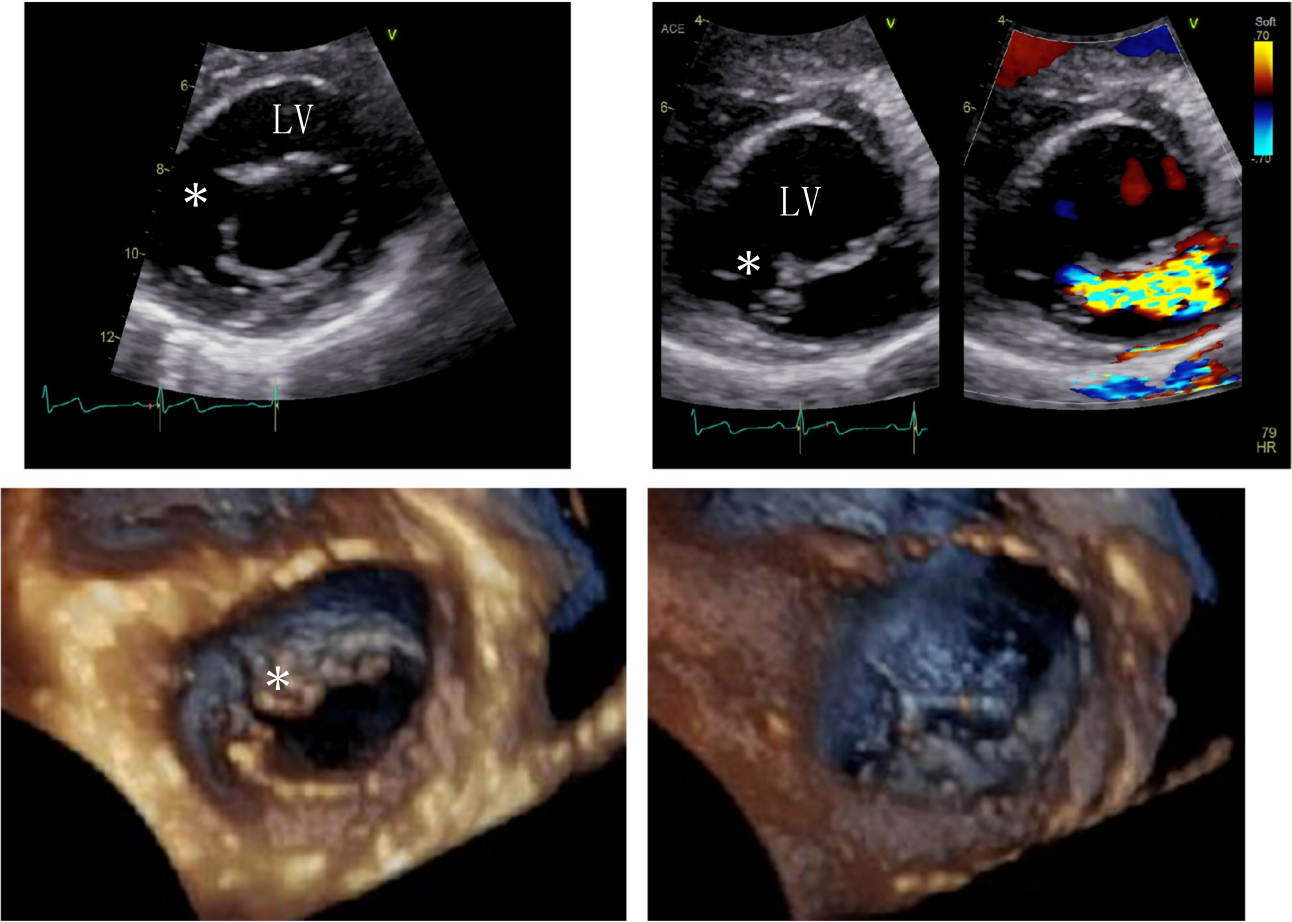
Two-dimensional transthoracic echocardiography (parasternal short-axis view) in a patient with isolated anterior cleft of the mitral valve (*). A mitral regurgitation jet is obtained from the isolated anterior cleft of the mitral valve leaflet
